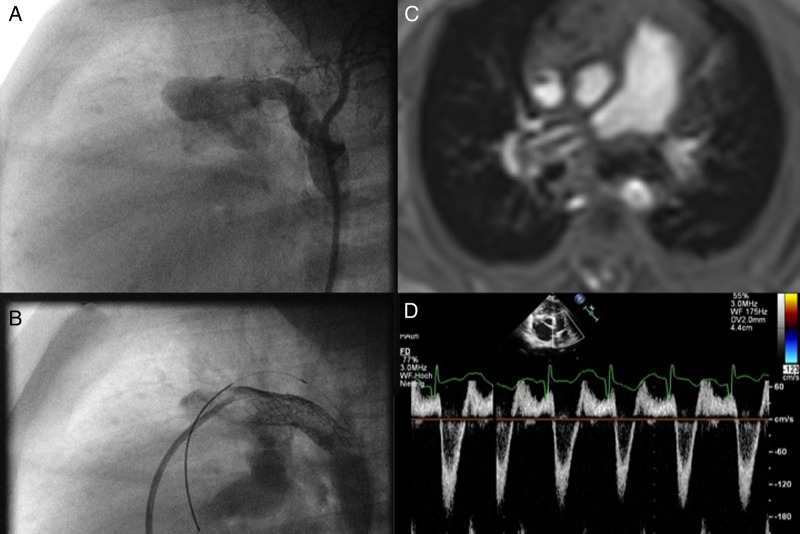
(A–D) The need for a valved Potts shunt. (A) shows a surgically performed reversed ‘Potts’ shunt with a dimension of 6 mm; in the follow-up, the polytetrafluorethylen (PTFE)-shunt was dilated by placing a 8 mm Formula Stent (B); but despite a bilateral pulmonary banding, which is depicted on MRI (C), the Doppler echocardiography (D) shows a systolic right-to-left, but a diastolic left-to-right shunt flow.
